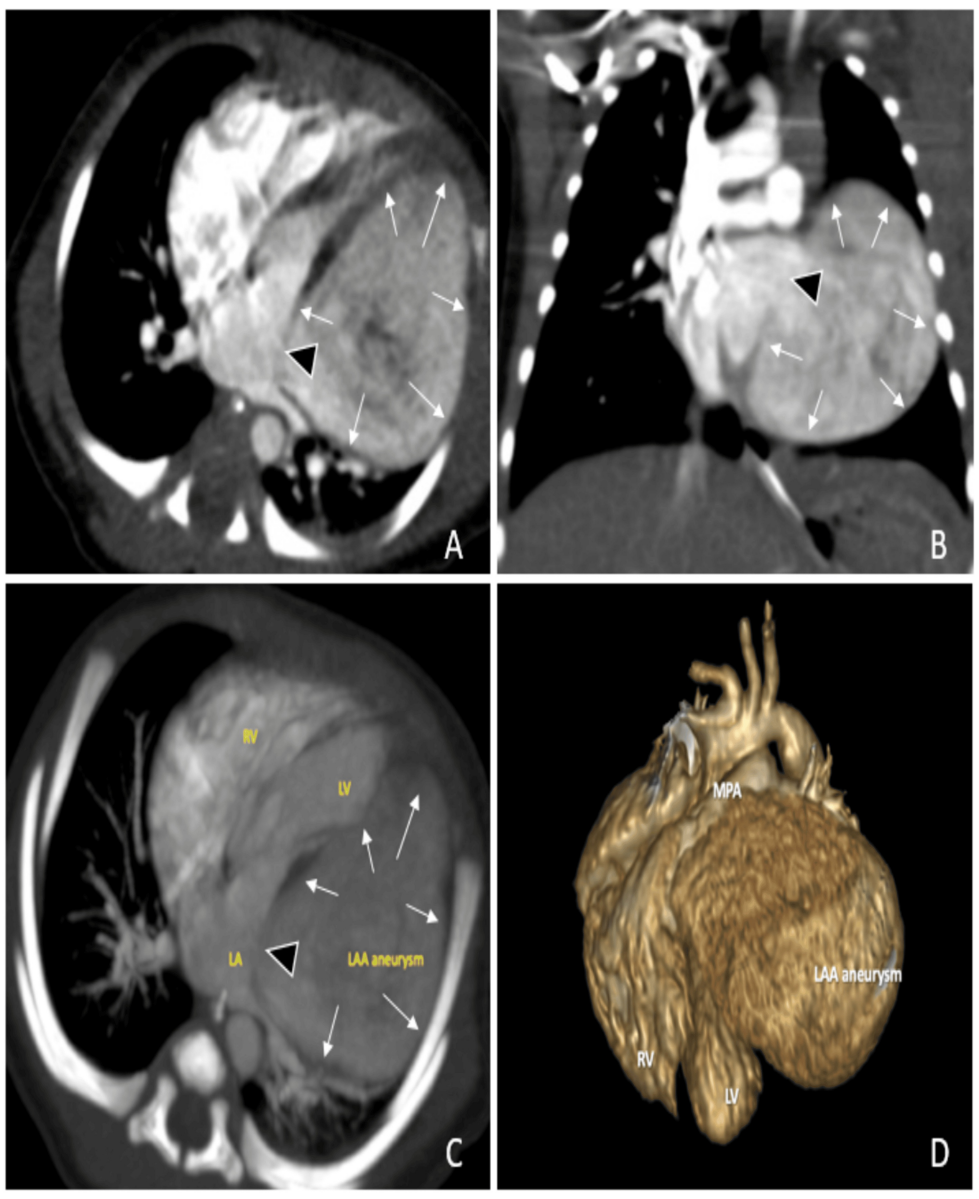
Computed tomographic angiography of the heart showed a single cystic mass adjacent to the left side of the heart. (A) Axial view, (B) coronal view, (C) maximum-intensity projection axial view, and (D) 3D reconstruction. LA: left atrium; LAA: left atrial appendage; LAAA: left atrial appendage aneurysm; LV: left ventricle; MPA: main pulmonary artery; RV: right ventricle; black arrow heads: communication of LAAA to LA (LAAA neck); small white arrows: extension of the LAAA
